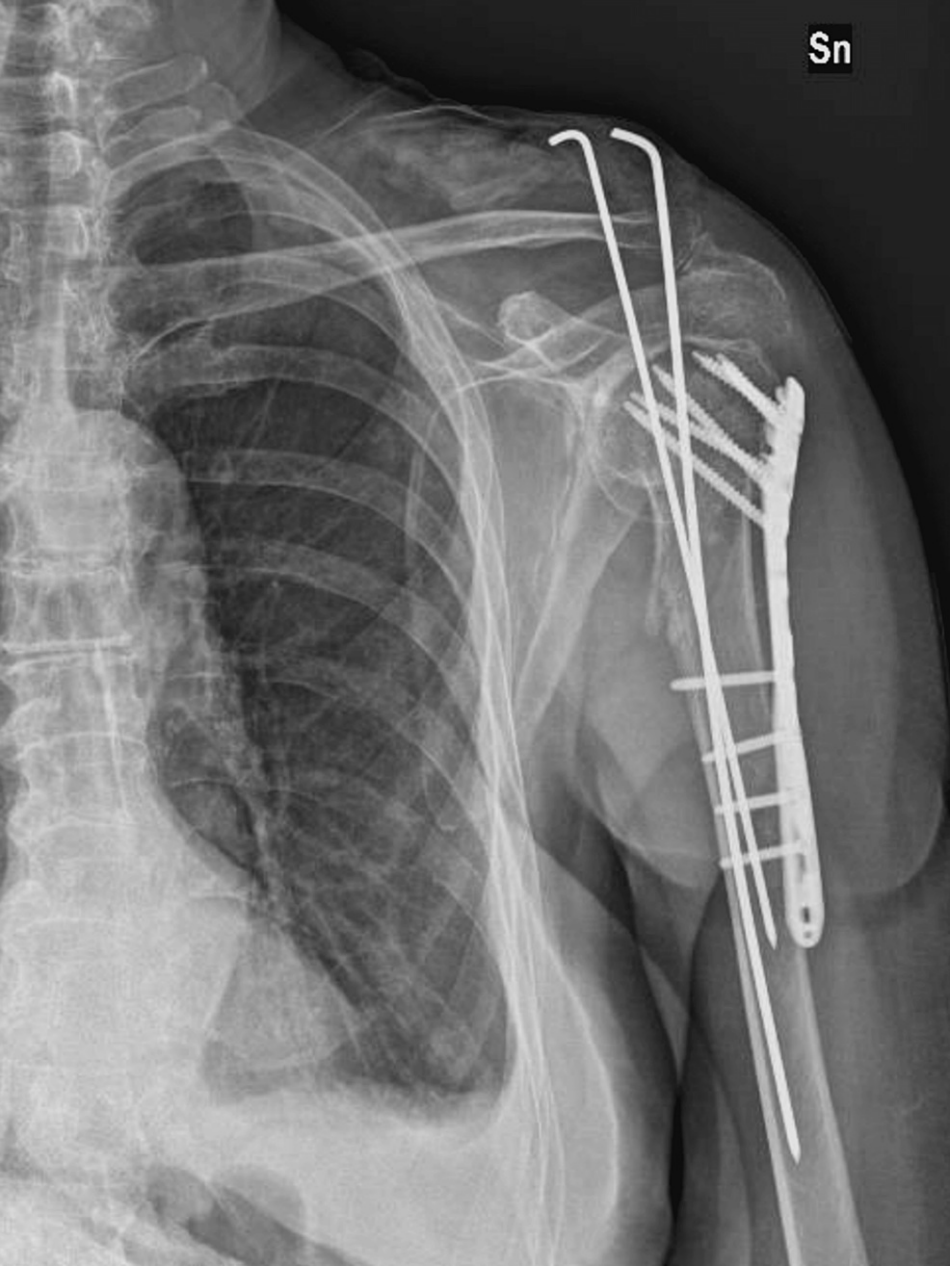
Post-surgical treatment open reduction internal fixation X-ray (Case 1) Sn: left
